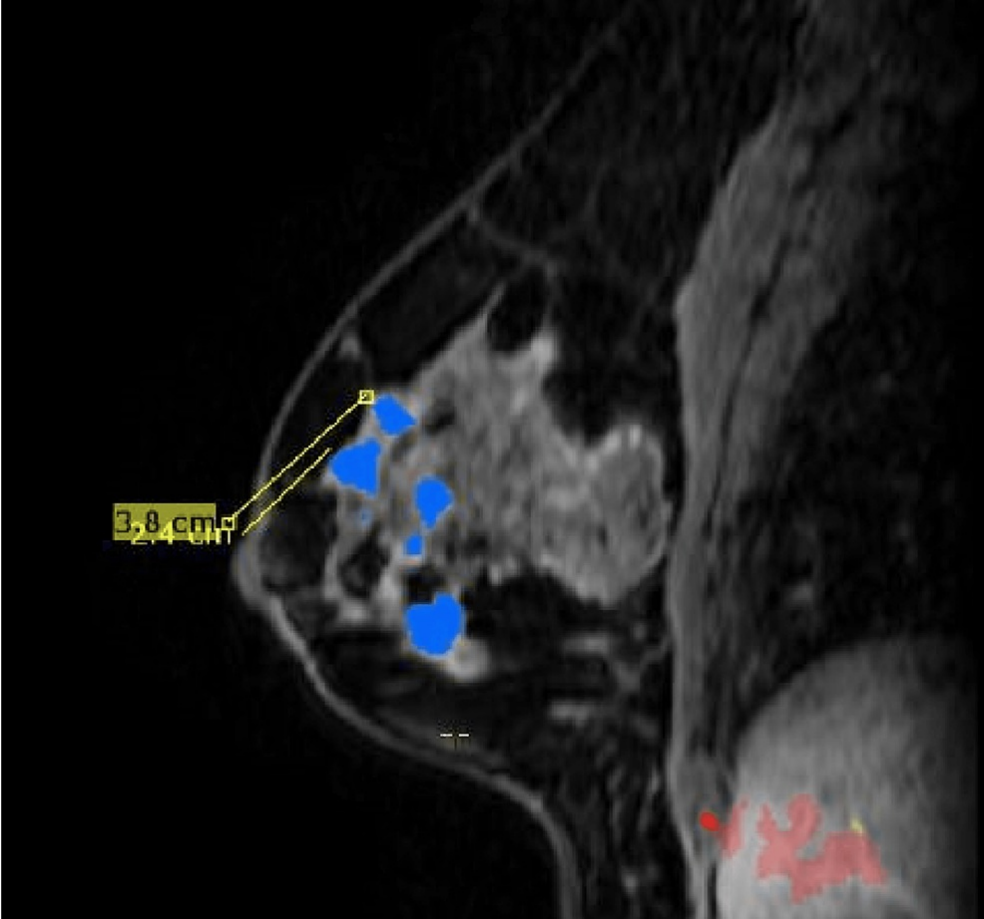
Additional masses found at one o'clock and 12 o'clock MRI findings stated that "At the one o'clock position, approximately 2.4 cm from the nipple, there is a rounded mass, measuring 0.8 cm. At the 12 o'clock position, approximately 3.8 cm from the nipple, there is a rounded mass, measuring 1 cm."
